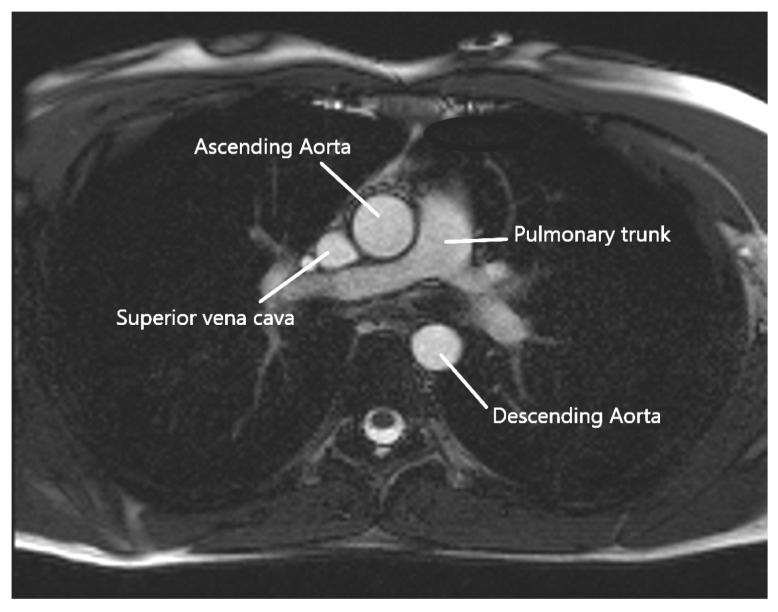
Axial MRI at the level of the pulmonary trunk.
